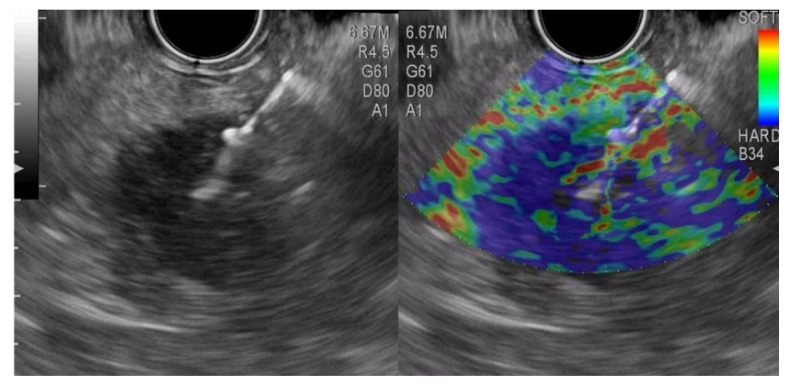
Elastography endoscopic ultrasound fine-needle aspiration from a pancreatic neuroendocrine tumor.
